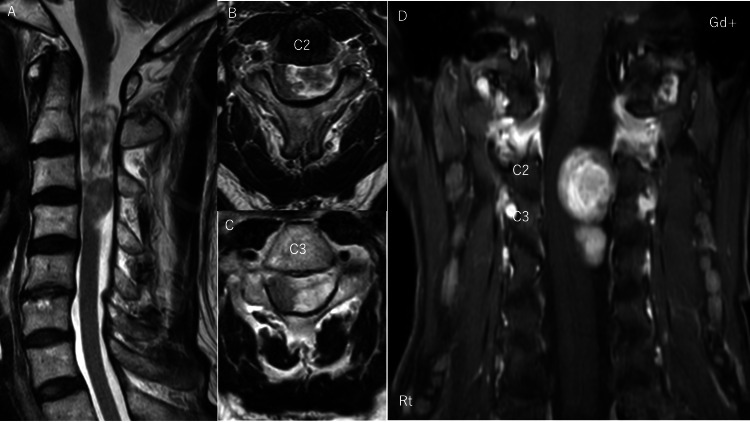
Preoperative MRI of case 2 (A) T2-weighted sagittal section showed the spinal cord tumor from C2 to the C4 vertebral body. (B) T2-weighted cross-sectional image at C2 level showed that the tumor occupied almost all of the dural canal. (C) T2-weighted cross-sectional image at C3 level showed that the tumor occupied more than half of the dural canal. (D) A gadolinium-enhanced coronal section showed that the spinal cord tumor was distributed to the left side.
